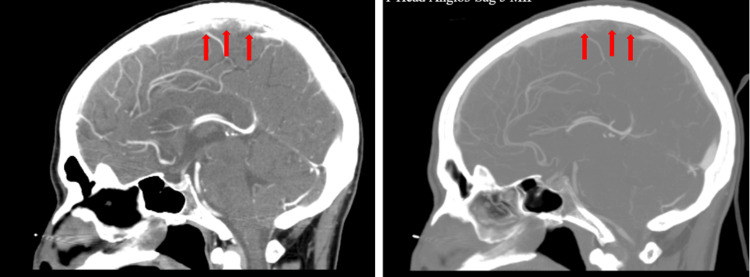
CT venogram showed multiple filling defects (red arrows) suggesting sagittal sinus thrombosis CT: computed tomography
